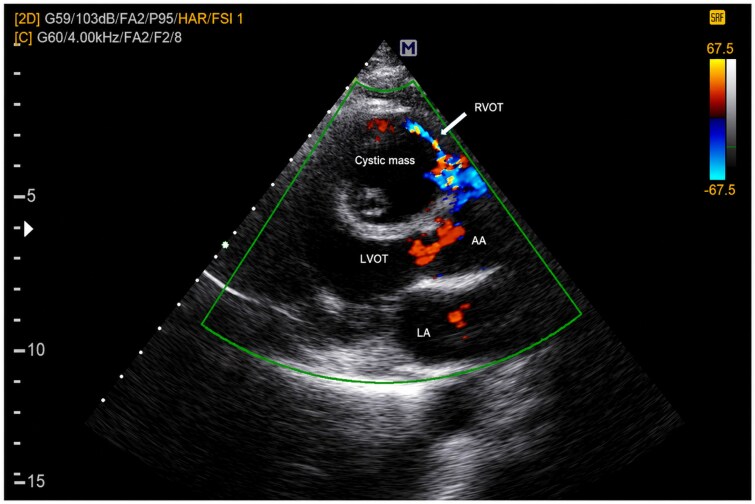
Colour Doppler transthoracic echocardiography (parasternal long-axis view) demonstrating turbulent flow across the right ventricular outflow tract secondary to protrusion of the interventricular septal hydatid cyst, consistent with severe right ventricular outflow tract obstruction.
